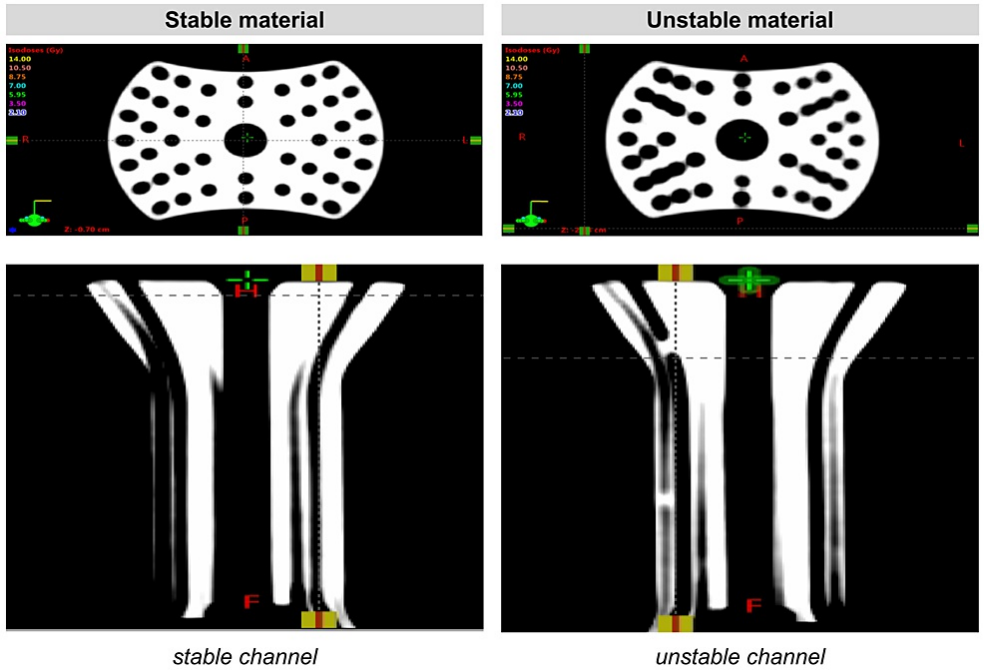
Comparing CT scans with needle trajectories of UFO device iterations printed in stable material to unstable material UFO: Universally Friendly Obturator.
